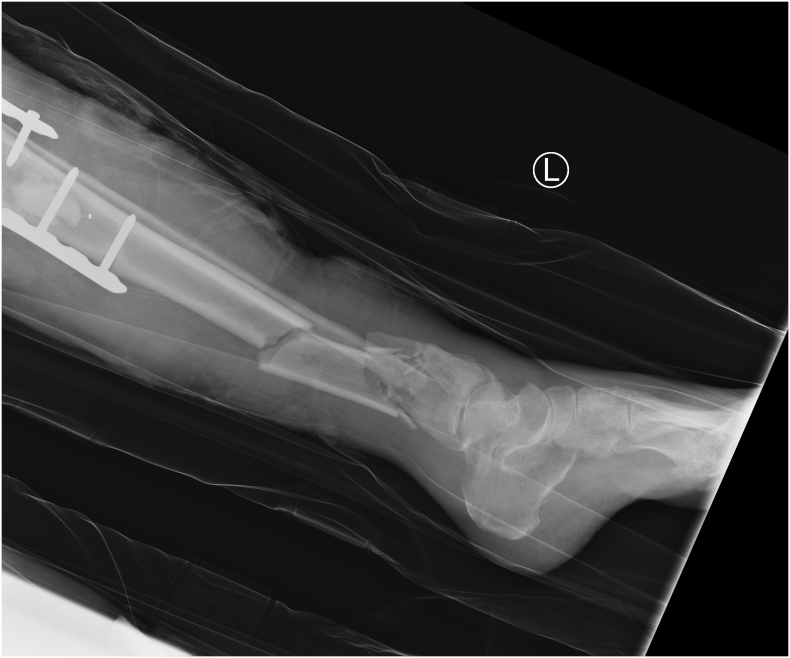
Lateral radiograph of the left leg showing a comminuted fracture of the tibia + fibula with metalwork from previous knee arthrodesis in situ.
